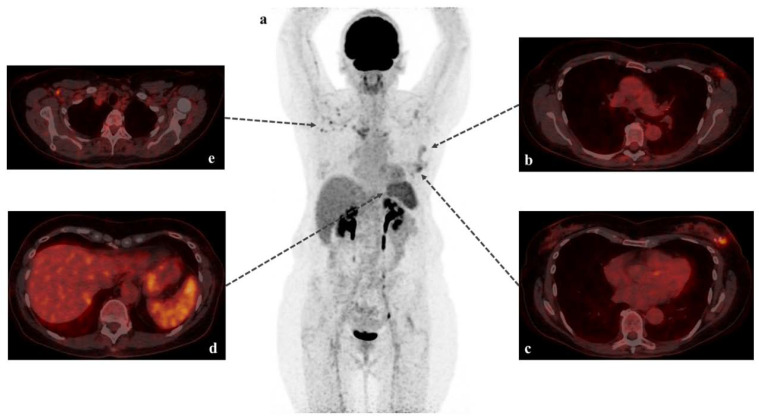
[18F]-FDG PET/CT scans with MIP (maximum intensity projection: (a) and fused axial images (b–e). Staging [18F]-FDG PET/CT revealed postoperative aspects in the left breast and ipsilateral axilla (b,c). Interestingly, we also found a diffusely high spleen glucose metabolism (d): maximum and mean standardized uptake value of 5.5 and 4.3, respectively), which was higher than the normal liver uptake (maximum and mean standardized uptake value of 4.4 and 3.2, respectively) frequently used as an internal reference organ to assess the significance of FDG uptake in pathologic processes. These findings were associated with hypermetabolic right axillary lymph nodes (e): maximum and mean standardized uptake values of 5.6 and 3.0, respectively). No pathological uptake of FDG was detected within the subcutaneous soft tissues of the right deltoid, corresponding to the injection site of the viral vector vaccine (AZD1222) [1].
